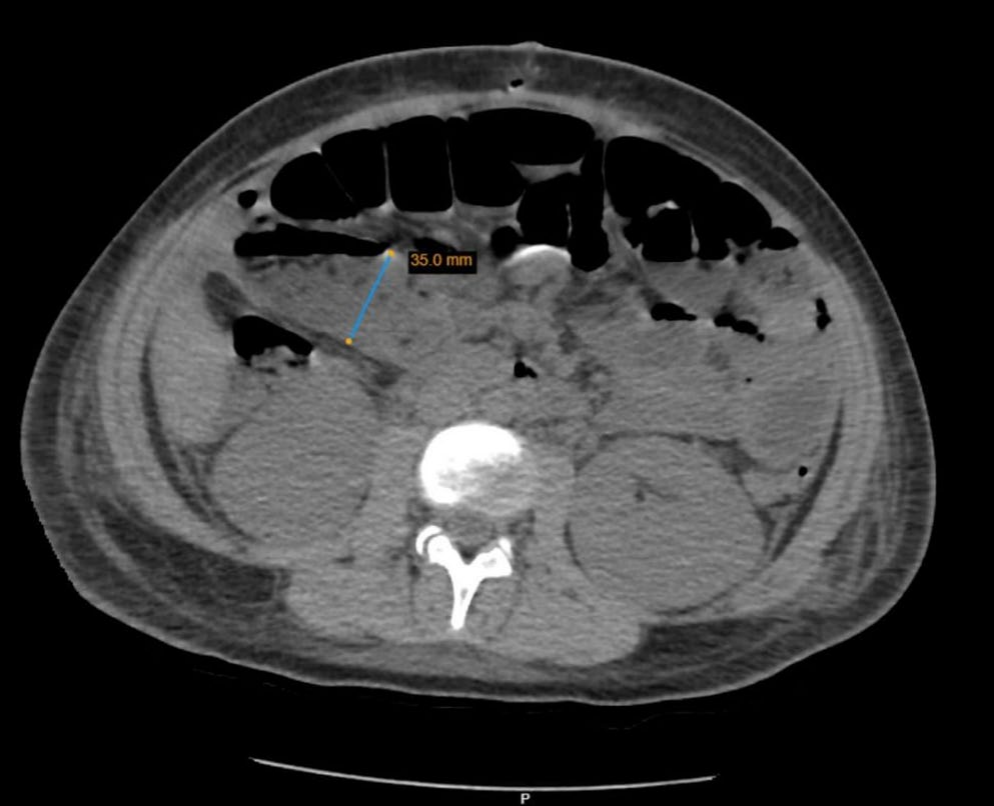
Abdominal CT scan of focal defect in the enhancing appendiceal wall, with extraluminal gas.
